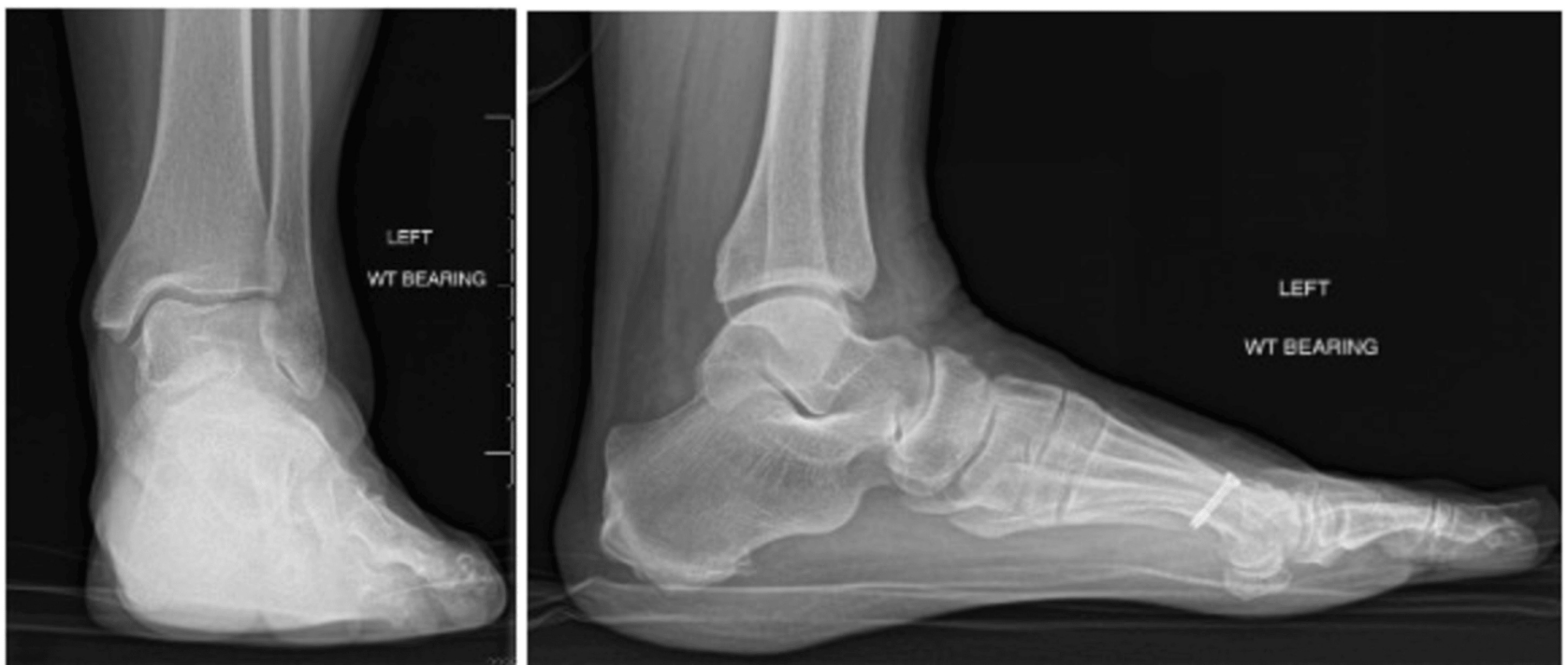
WB X-ray 13 months post-inciting event and approximately seven months after the development of AVN. WB: weight bearing; AVN: avascular necrosis
